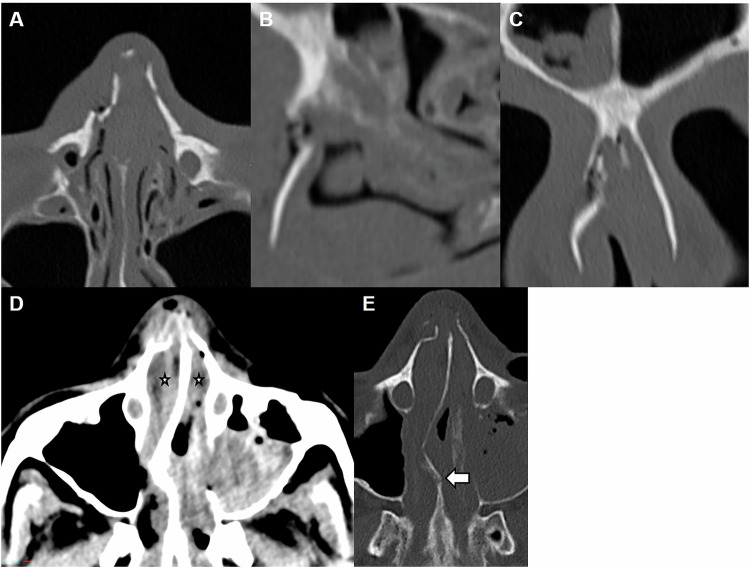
Facial fractures. Axial (A, E), sagittal (B) and coronal (C) bone window and axial soft tissue window (D) CT images of the nasal bone and septum revealing a dislocated fracture of the right nasal bone (A–C). The lower images show a displaced nasal bone and septum fracture (E) as well as a bilateral nasal septal hematoma (star in D)
